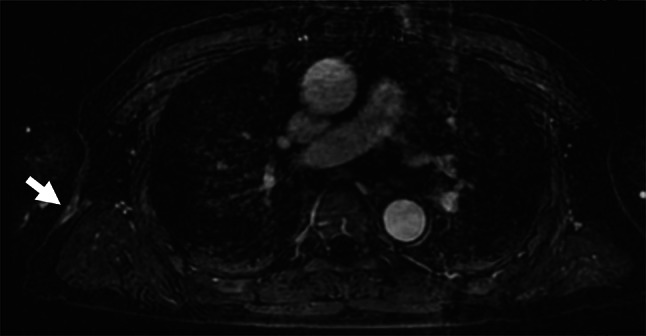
After extirpation of the mass, MRI showed only slight enhancement at the scar site, with no significant local residual tumor (arrow)
